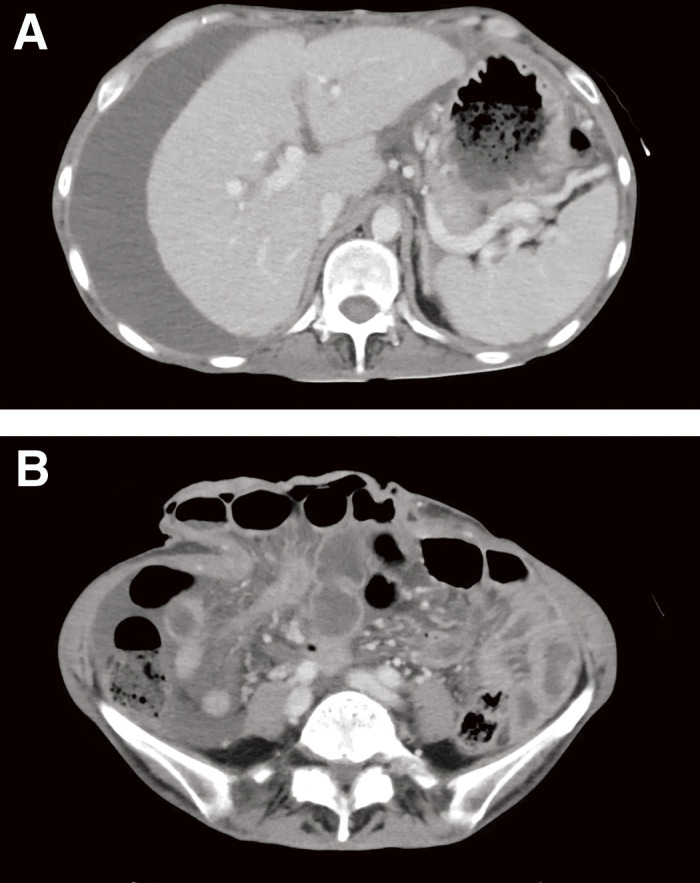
(A) CT scan displaying signs of liver cirrhosis, massive ascites, and hepatic atrophy. (B) A midline incisional hernia with a diameter of 10 cm and protrusion of the small intestine and transverse colon.
